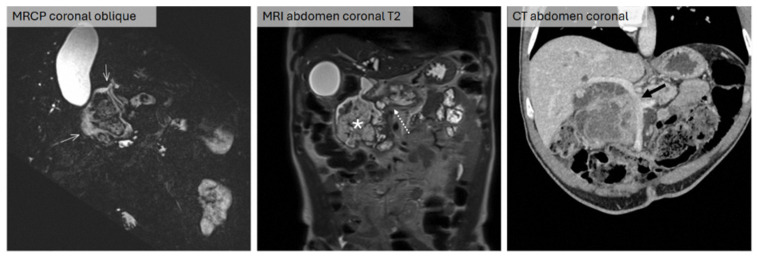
(Left) Surveillance MRI abdomen (coronal oblique, 0.6 mm SSFP sequence) shows multiple fistulas between the mass and the proximal duodenum (white arrows). (Middle) Same MRI abdomen (coronal T2 sequence) showing dilated MPD (white dashed arrow) with multiseptated T2 hyperintense mass lesion in the pancreatic head compatible with IPMN (white asterisk). (Right) Contrast-enhanced CT abdomen (coronal) showing involvement of the adjacent SMV and portal vein (black arrow).
